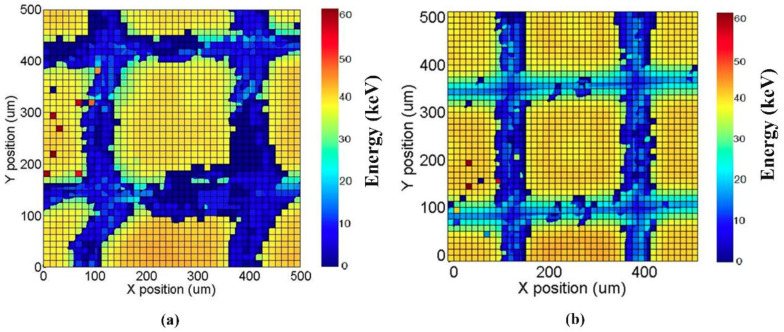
The results of a 2D synchrotron X-ray mapping of the small 250 μm array at 40 keV, for (a) the LF-CZT and (b) HF-CZT detectors. The changes in the photopeak energy are confined near the inter-pixel gaps because of charge-sharing effects, which are more severe for the LF-CZT detector.
